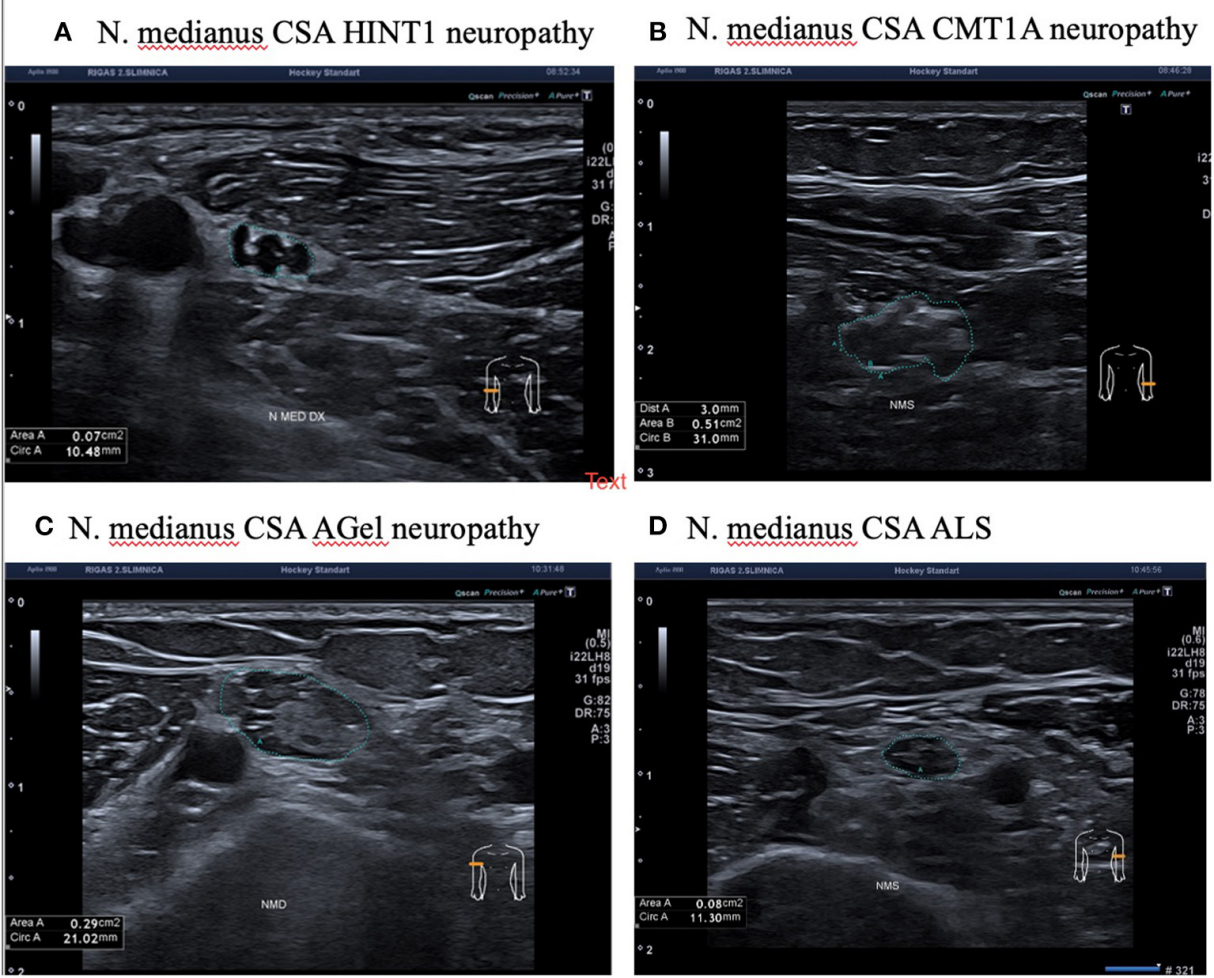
Median nerve ultrasonography in patients with HINT1 neuropathy, CMT1A, ALS, and AGel. (A) N. Medianus CSA *HINT1* neuropathy. (B) N. Medianus CSA CMT1A neuropathy. (C) N. Medianus CSA AGel neuropathy. (D) N. Medianus CSA ALS.
